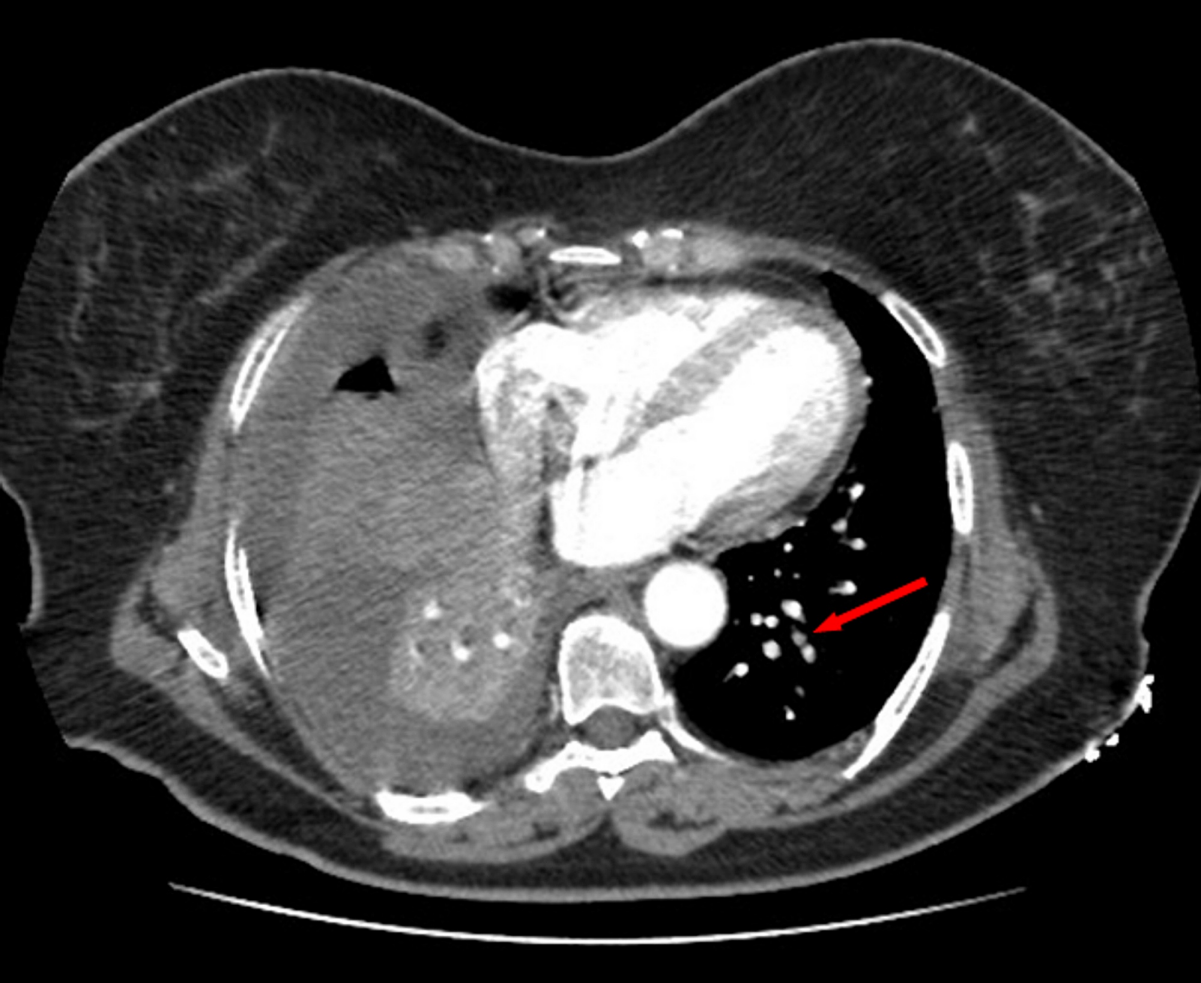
Computed tomographic pulmonary angiography showing a distal pulmonary embolism
